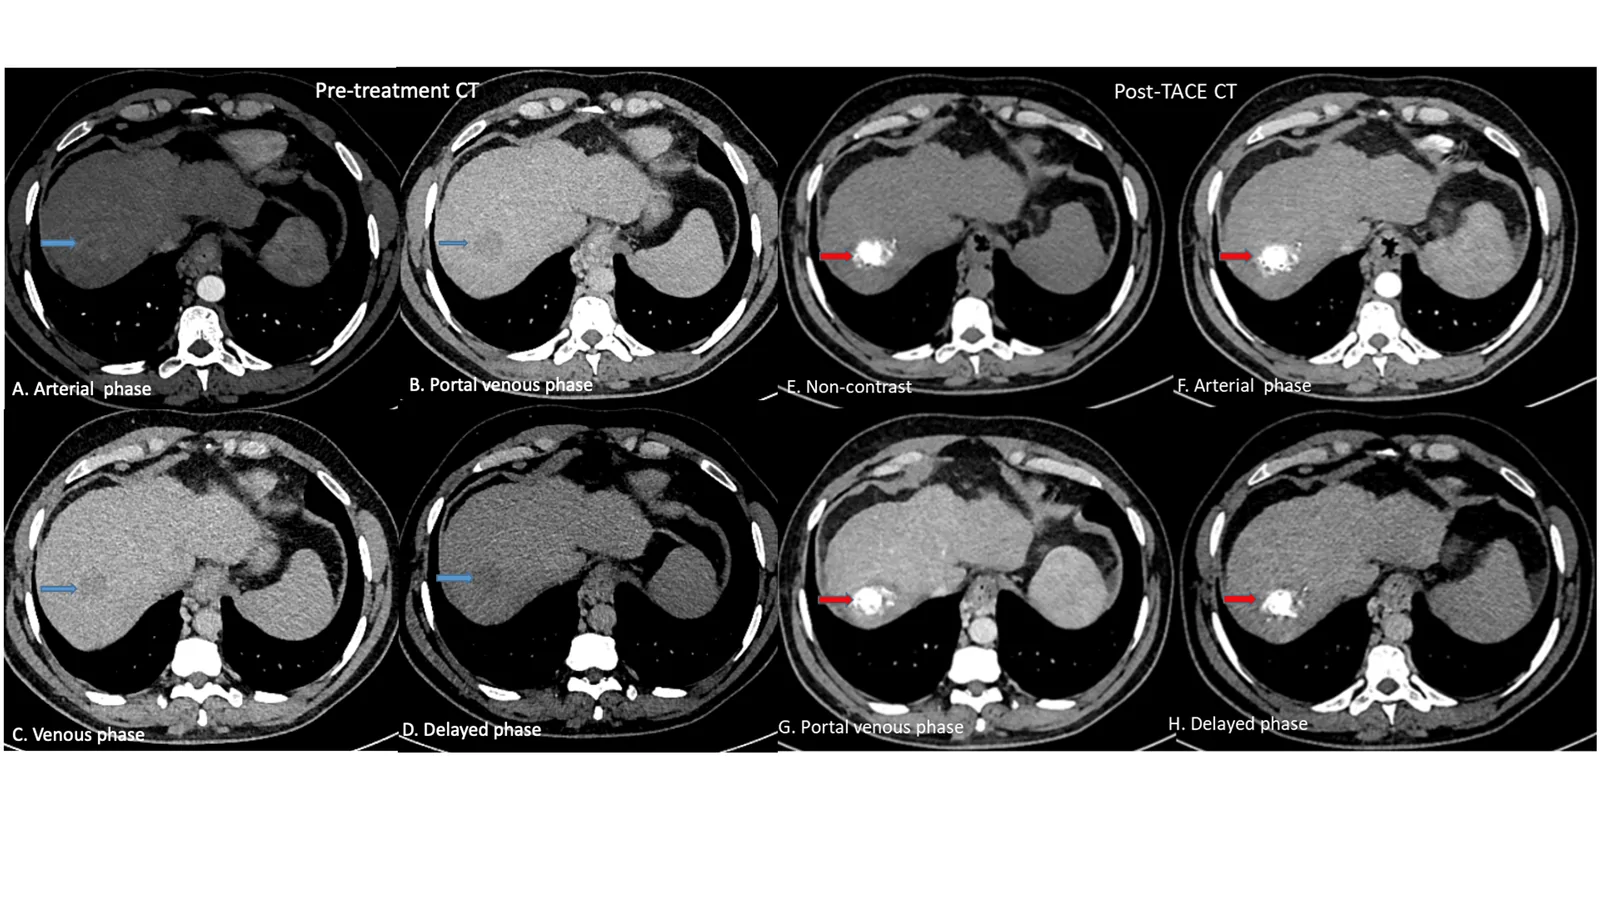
LR-TR nonviable category after conventional TACE on multiphasic CT. Pretreatment axial multiphasic contrast-enhanced CT images (A-D) obtained during the arterial (A), portal venous (B), venous (C), and delayed (D) phases demonstrate a well-defined 17-mm hepatic observation in segment VIII (blue arrow). The observation demonstrates nonrim arterial phase hyperenhancement (A) and nonperipheral washout on the portal venous, venous, and delayed phases (B-D), consistent with an LR-5 observation. Post-treatment axial non-contrast and multiphasic contrast-enhanced CT images (E-H) obtained after conventional transarterial chemoembolization (TACE) during the non-contrast (E), arterial (F), portal venous (G), and delayed (H) phases demonstrate dense lipiodol deposition at the treatment site (red arrow) without nodular, mass-like, or thick irregular arterial phase hyperenhancement within or along the margins of the treated observation, consistent with an LR-TR Nonviable category.
